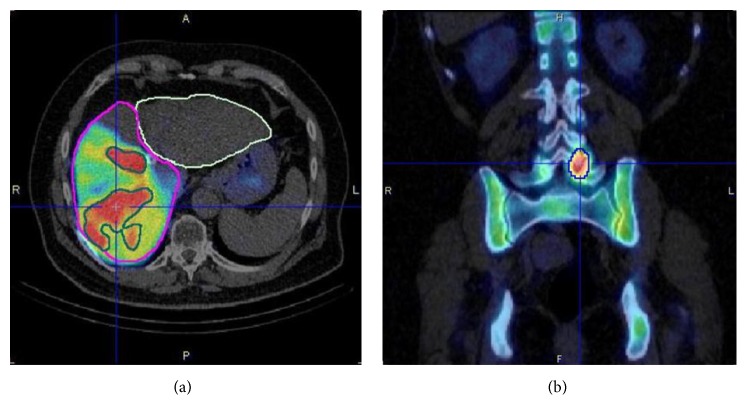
(a) ^99m^Tc-MAA SPECT/CT prior to Y-90 radioembolisation after administration of 105.8 MBq of ^99m^Tc-MAA to the right lobe of the liver (volume = 1290 mL, segmentation in violet). In this specific case the recovered total activity on the SPECT field of view was 98.2 MBq that is 7% less than the total administered activity. Average activity concentration in the normal parenchyma was 35.6 kBq/ml (range: 0–151 kBq/ml, volume = 987 mL). In the region of high uptake (typical of tumor hypervascularisation, volume = 303 mL, segmented in green) the averaged activity concentration was 107 kBq/ml (range: 49–421 kBq/mL). (b) Bone SPECT/CT of a patient administered with 937 MBq of ^99m^Tc-labeled diphosphonates. A hot lesion due to a degenerative arthrosis is visible on the left lumbar posterior vertebral articulation (L5-S1) with average activity concentration of 224.5 kBq/mL (range: 172–328 kBq/mL) with an average bone uptake into the SPECT field of view of 60 kBq/mL (SUV_max_ = 46 g/mL).
